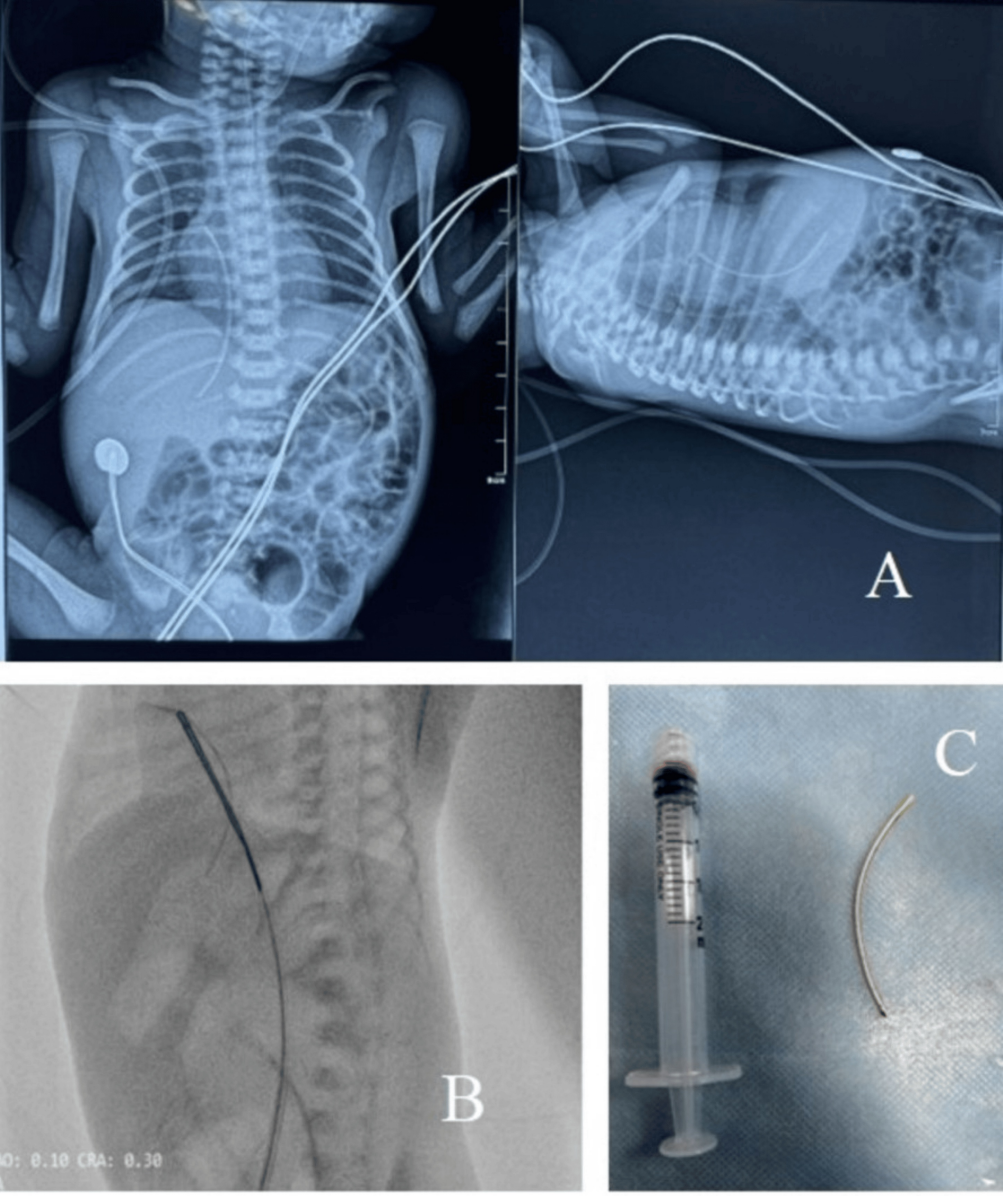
(A) Anteroposterior (AP) and lateral chest radiograph showing the stuck umbilical vein catheter in the right atrium (RA) and inferior vena cava (IVC). (B) Fluoroscopy showing the catheter being snared out using femoral access. (C) Removed broken fragment of the umbilical vein catheter.
